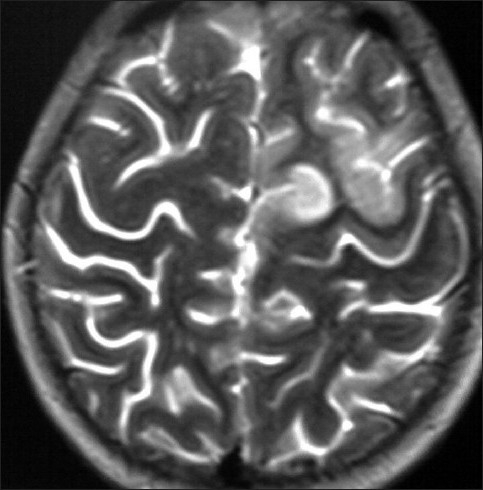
T2-weighted axial MR image showing a small area of subcortical white matter edema in the left high parietal parasagittal region due to a venous infarct
